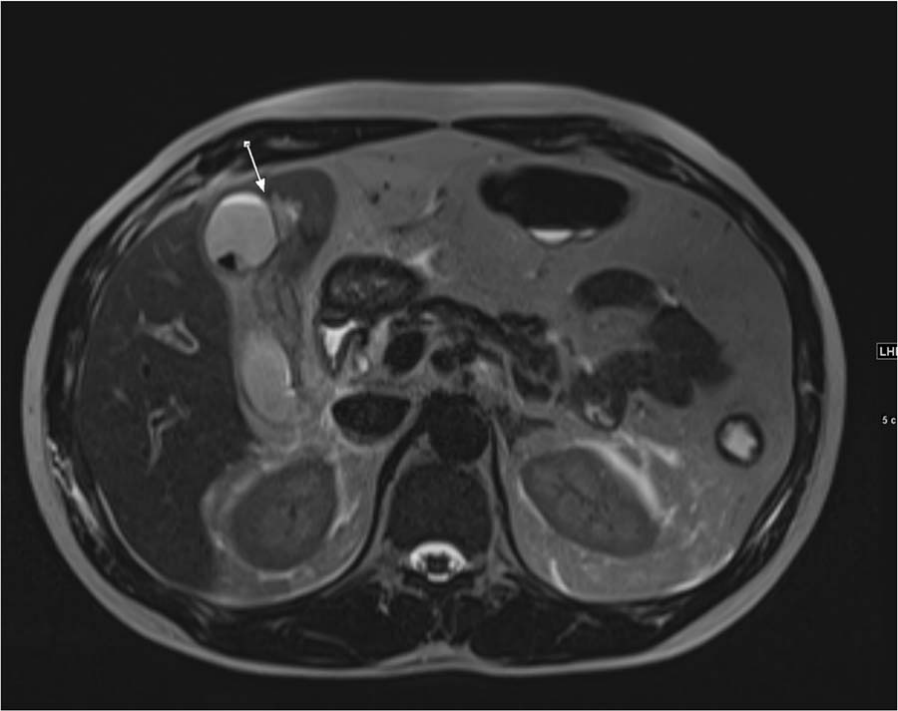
Axial cut MRCP showing T2-weighted images. Arrow indicates area of micro-perforation and small collection.
